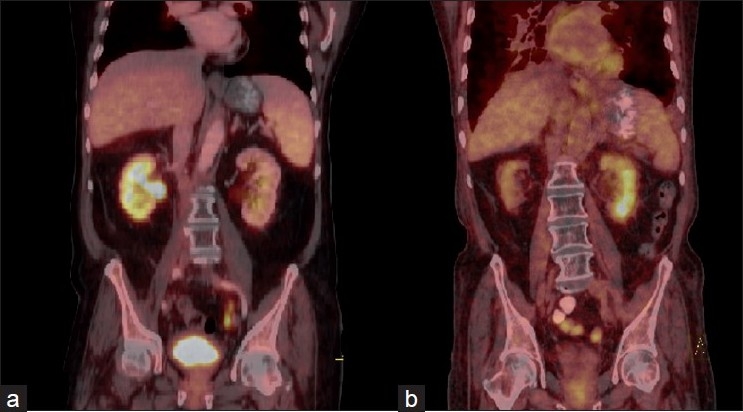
Review of pre- and post-therapy PET CT studies of a 71-year-old male patient, a known case of abdominal non–Hodgkin’s lymphoma, revealed significant reduction in size and metabolic activity of retroperitoneal lymphomatous lesion. Increased FDG activity in the corticomedullary space of right kidney in pretherapy study (a), reported as lymphomatous involvement, was not seen in the subsequent scan which showed decreased size. Serum creatinine levels showed increase in values from 0.8 to 2.3 during the interval between these studies, for which a non-contrast follow-up PET/CT study was done.- In follow-up PET/CT study, previously normal appearing left kidney showed intense corticomedullary tracer activity along with associated surrounding fat stranding and thickened perirenal fascia bilaterally (b). Above findings suggested inflammatory/pyelonephritic nature of the disease, thus impressing upon the importance of early detection of inflammatory etiology of the kidney and differentiating it from malignant involvement while reporting PET/CT cases showing increased corticomedullary renal uptake.
